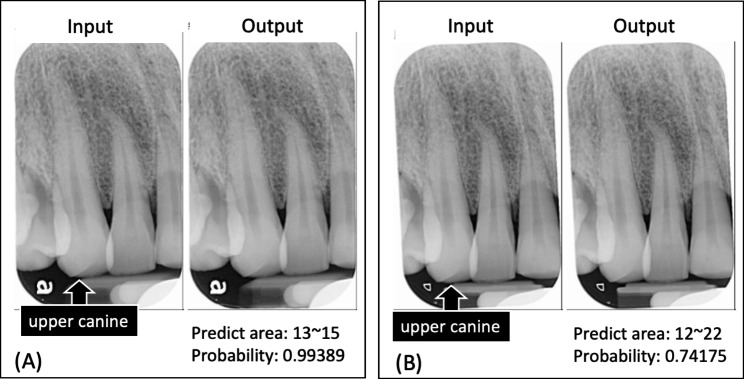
Example of an intraoral film that was incorrectly classified by the AI model. (A) When the operator takes the X-ray with a horizontal tilt angle of 0 degrees, the AI model correctly recognizes the desired tooth area to be captured. However, when the tube tilts from the medial to distal by 15 degrees (B) The projected image resembles the capture angle of teeth 12–22, leading the AI model to categorize it into the 12–22 tooth area
